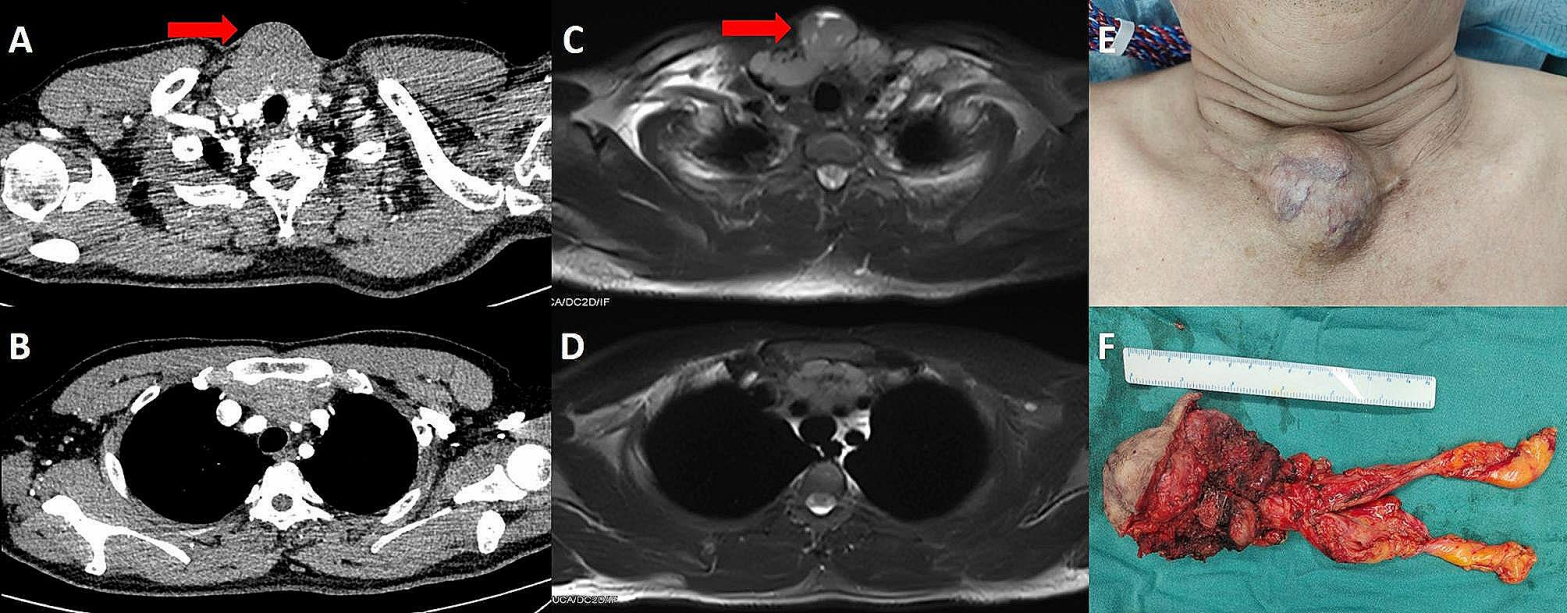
A and B: Plain and contrast-enhanced neck and chest CT revealed irregular mass shadow was observed in the anterior neck, posterior to the sternum, which protruded noticeably from the surface. The mass had a relatively large cross-sectional area of approximately 6.3*4.6 cm. C and D: Enhanced MRI of neck and chest showed that the mass with T1 iso-intensity and T2 high-intensity signals was observed in the anterior neck and upper anterior mediastinum, posterior to the sternum. On DWI, it showed high-intensity signals and had a size of approximately 6.8 × 4.8 × 6.5 cm. The mass showed uneven enhancement and contained small patchy short-T1 and long-T2 signal shadows. E and F: A mass was observed in the anterior neck and it was resected completely
